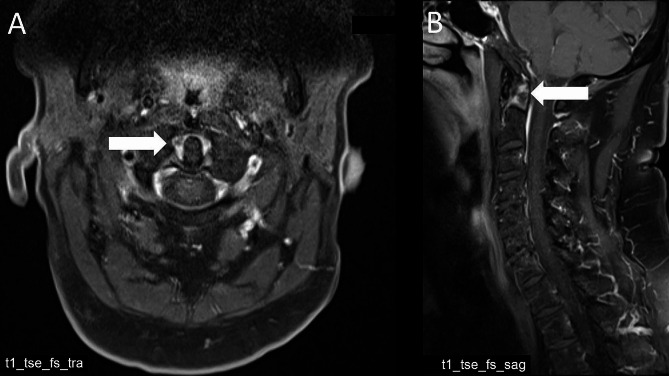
Atlantodental synovitis. a Transversal and b sagittal MRI (T1- weighted with contrast agent), showing atlantodental synovitis (arrow) of a patient with rheumatoid Arthritis
